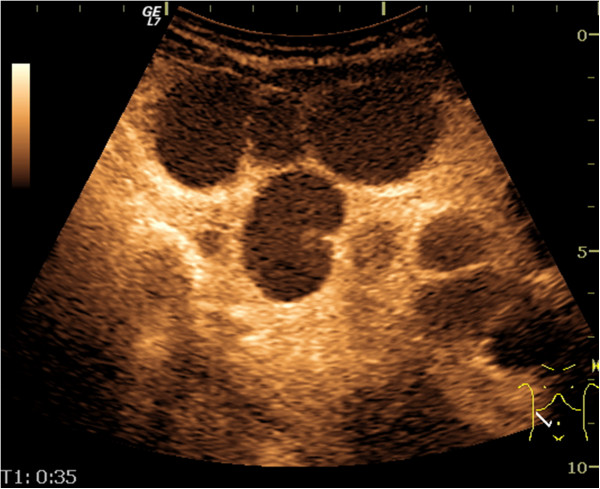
Contrast-enhanced ultrasound - venous phase (35 seconds) – cystic mesenteric masses - no was/out of contrast agent.
